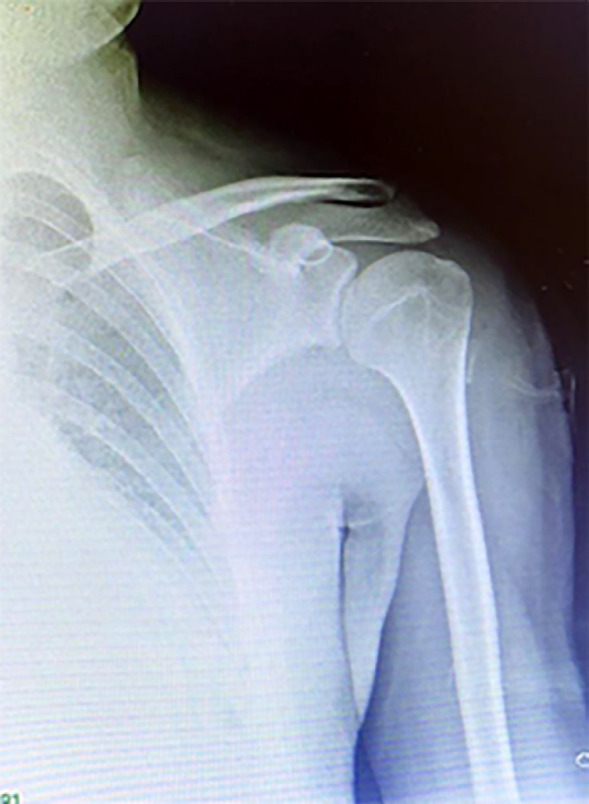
24-hour postoperative anteroposterior humerus radiography showing no bone lesions
